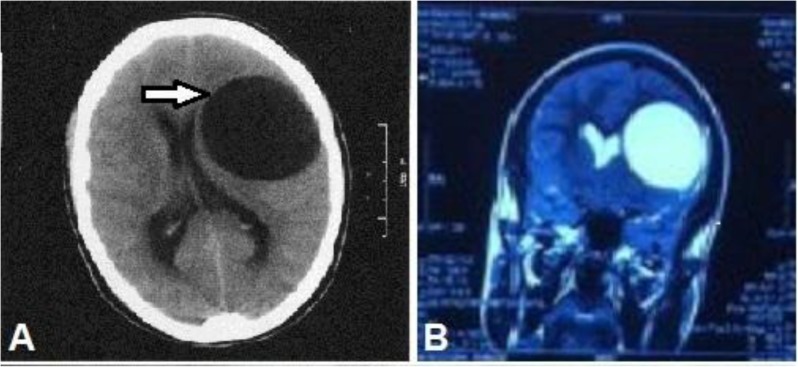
(A) Cerebral CT scan, cystic mass that measured 55 × 57 mm in the superior portion of left temporal lobe (B) Magnetic resonance imaging of brain that showing a large cyst lesion in the superior portion of left temporal lobe
